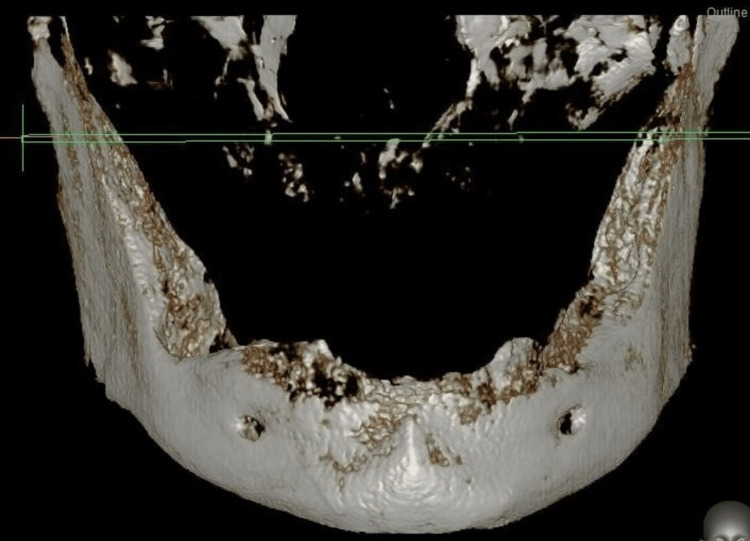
3D scanned image of the human mandible with Cone-beam CT-Vatech Smart Plus
